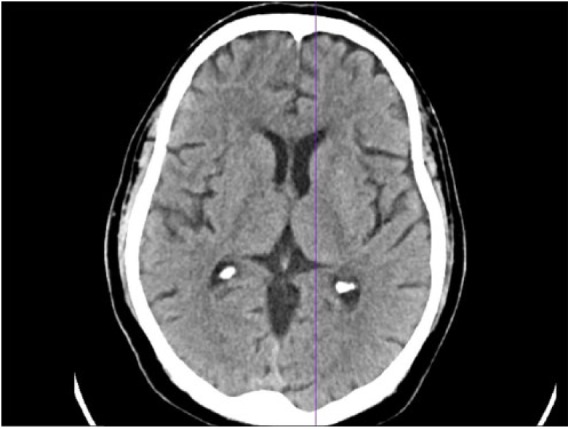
Computed tomography scan of the head showing normal imaging.
